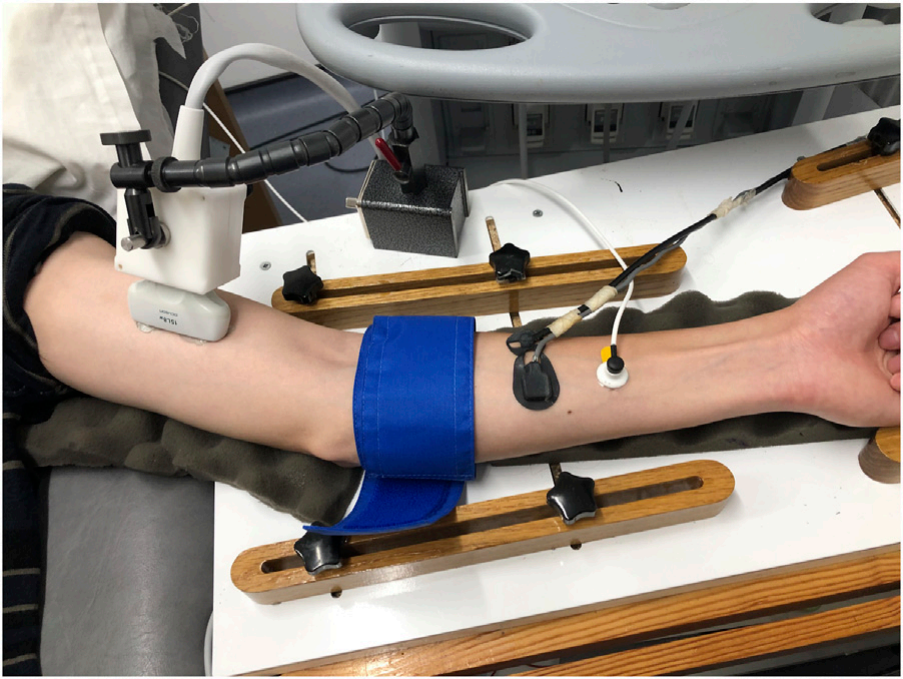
Representative image of placement of different probes and pneumatic cuff on the left arm. From left to right: ultrasound probe, pneumatic cuff, NIRS probe, laser Doppler probe.
